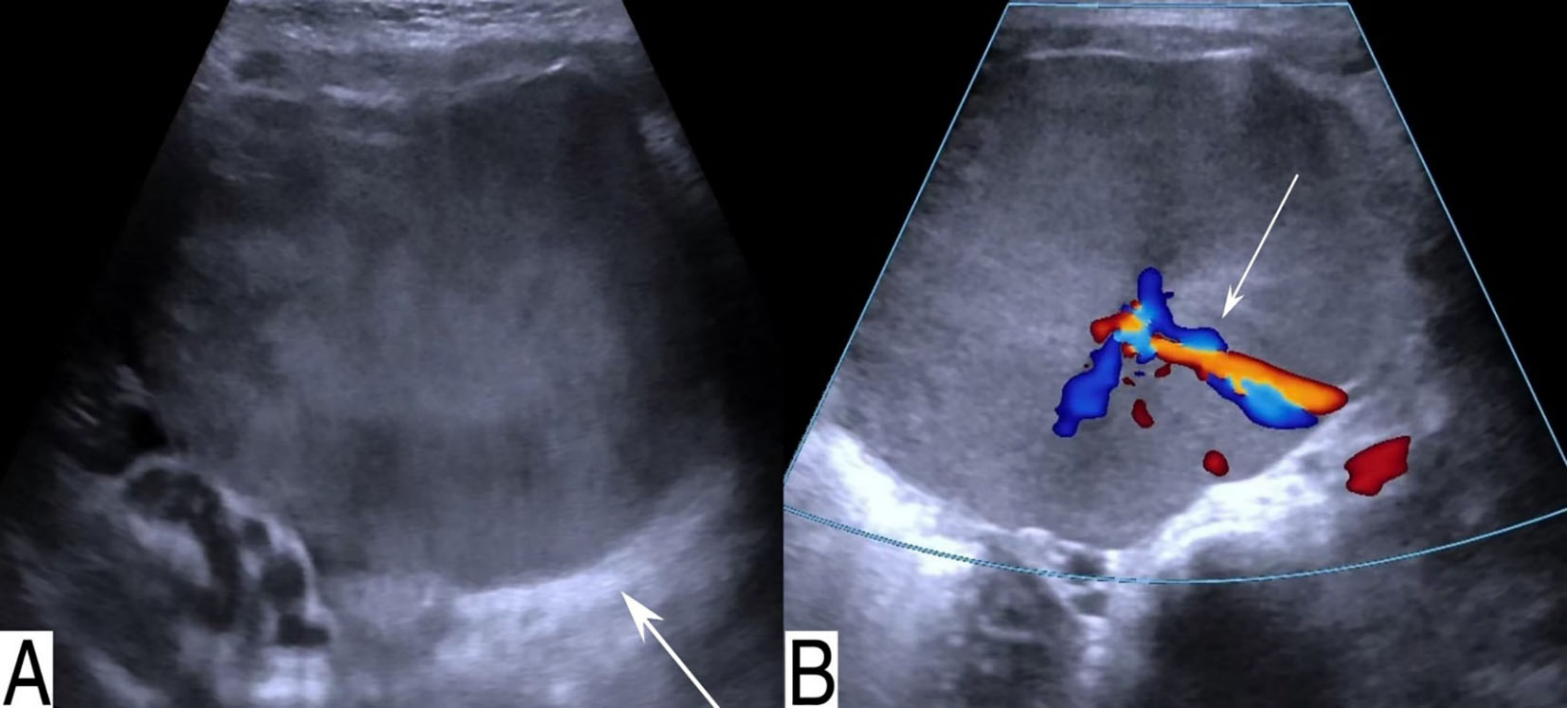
Conventional ultrasound. (A) Transverse ultrasound image shows a mass with mixed moderate-to-low echogenicity within the cavity (white arrow), displaying regular contours and clear boundaries. (B) Color Doppler imaging reveals short, strip-like blood flow signals within the lesion (white arrow).
